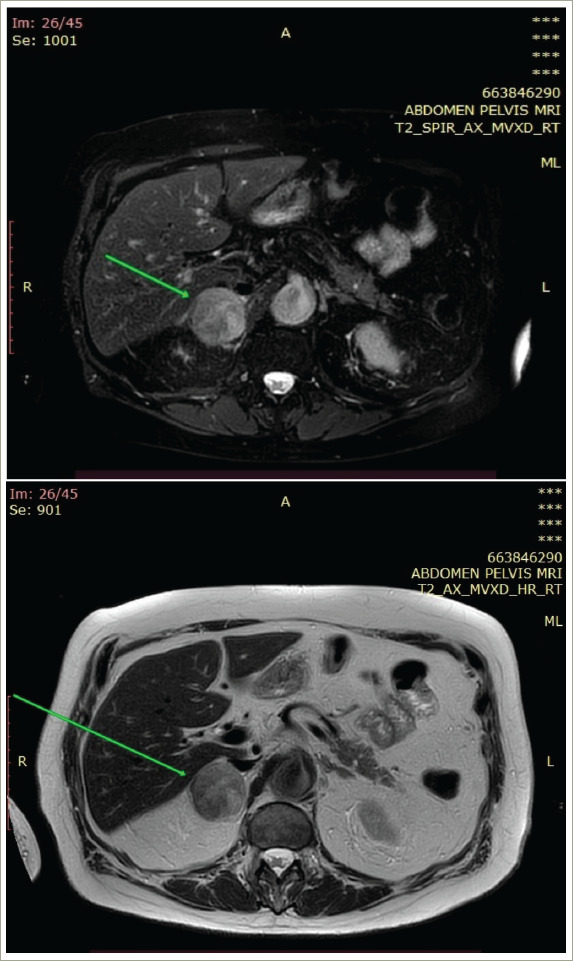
Magnetic resonance imaging depicting the right adrenal mass (arrow)
